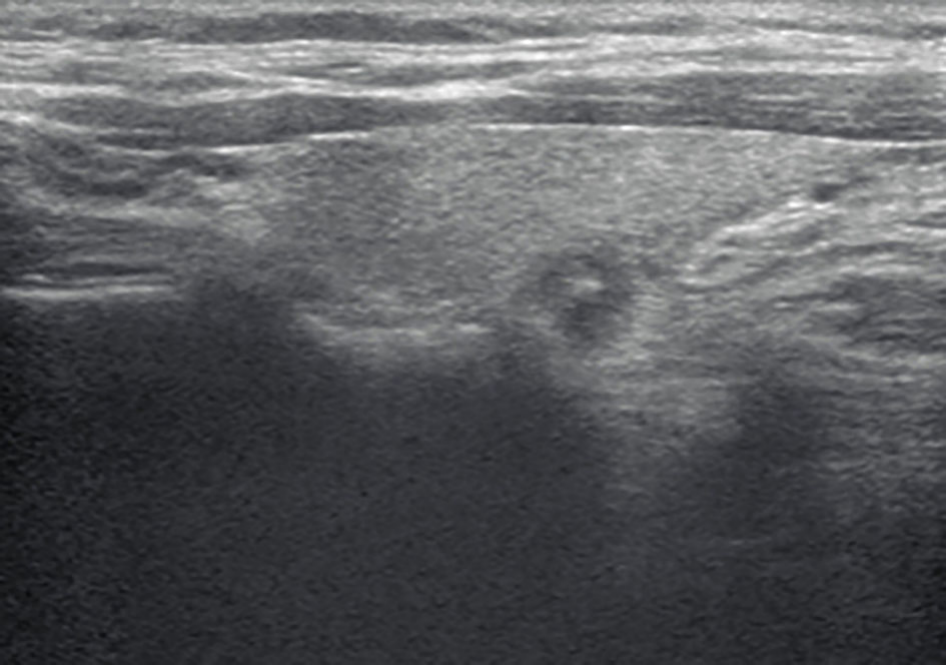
A longitudinal grayscale ultrasound (US) image obtained from a 37-year-old woman with papillary thyroid carcinoma shows a 7-mm solid, hypoechoic, and ill-defined margin thyroid nodule with punctate echogenic foci. This nodule was classified as TR4C according to the Kwak and C thyroid imaging reporting and data system (TIRADS). The original TIRADS does not recommend biopsy, while the modified TIRADS suggests biopsy.
